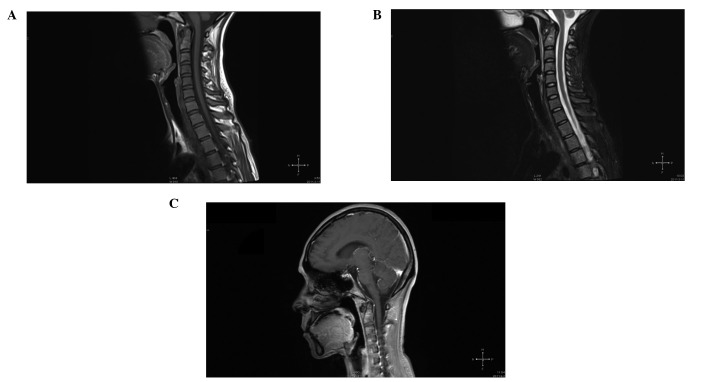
Follow-up cervical spine MRI obtained at 3 months after radiotherapy; (A) T1-weighted and (B) T2-weighted sagittal MRI. (C) Follow-up cervical spine MRI obtained at 9 months after radiotherapy showing permeative stablility of C1; T1-weighted Sagittal MRI. MRI, magnetic resonance imaging.
